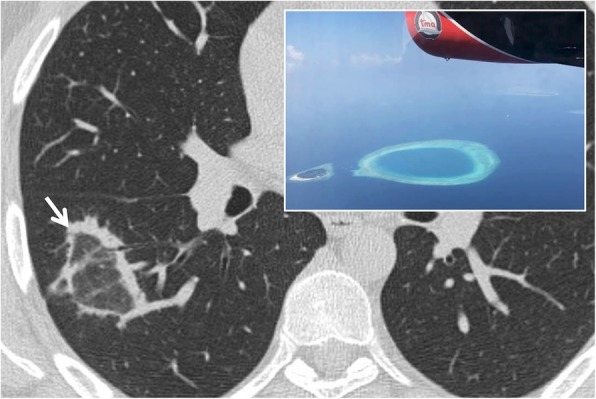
Atoll sign. The so called “atoll sign” (white arrow) is represented by a central ground glass area bordered by a peripheral consolidation. This CT finding—similar to the oceanic atoll—is frequently observed in patients with COP; it has been also called “reversed halo sign”
